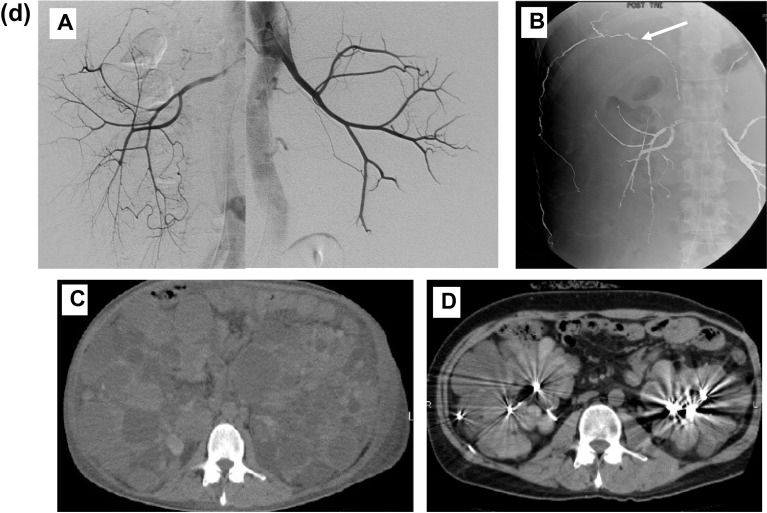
a Computed tomography (CT) 3 months before transfer to our hospital showed many areas suggestive of active renal hemorrhage (high density area with irregular border, black arrow) or old hemorrhage (higher density area, white arrow) in both kidneys. b Computed tomography showed generalized ventral subcutaneous free air including pus-filled fecal discharge (arrow) from the head to the lower abdomen. A, head; B, neck, C; upper abdomen (small arrow indicates the cystic (suggestive of diverticulum) fistula between the descending colon and the subcutaneous area); D, lower abdomen; E, coronary image of CT shows repeated cyst hemorrhage (high densitry area, black arrow) and subcutaneous free air including pus-filled fecal discharge (white arrow). c Enterography shows leakage of contrast medium from the descending colon (large arrow) into the subcutaneous area (small arrow). The perforation site was dilated and appeared to correspond to the diverticulum (black arrow). d Results of imaging and procedures. A, Renal artery angiography shows well-developed and stretched renal artery branches. B, Renal artery embolization was performed with a microcoil (arrow). C, Computed tomography before renal artery embolization. D, Computed tomography 12 months after renal artery embolization
